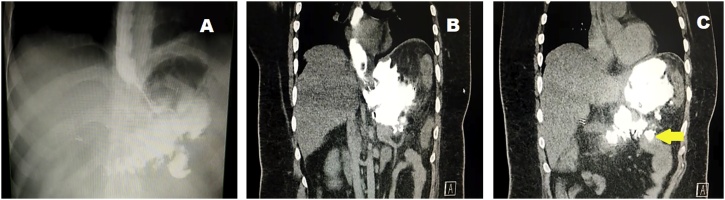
A: Preoperative water-soluble study showing leaking of contrast outside the stomach. B, C: Preoperative CT abdomen with IV and oral contrasts confirming presence of leakage with complete collapse of the stomach. The yellow arrow showing an area of suspicion.
